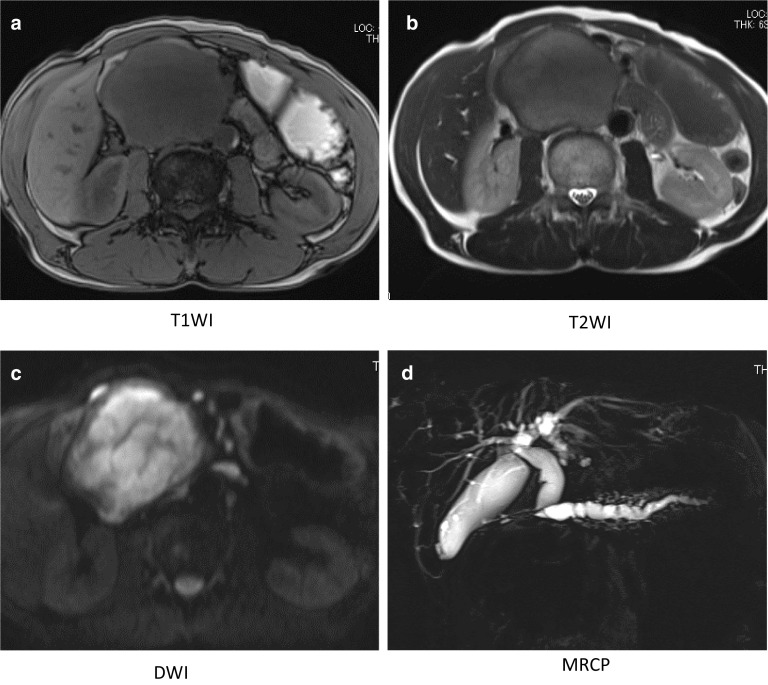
Abdominal MRI. MRI shows the tumor in the pancreatic head, with low signal intensity on T1-weighted imaging (a), low signal intensity on T2-weighted imaging (b), and high signal intensity on diffusion-weighted imaging (c). MR cholangio-pancreatography showing dilatation of intra- and extra-hepatic bile ducts and the pancreatic duct owing to tumor obstruction (d)
